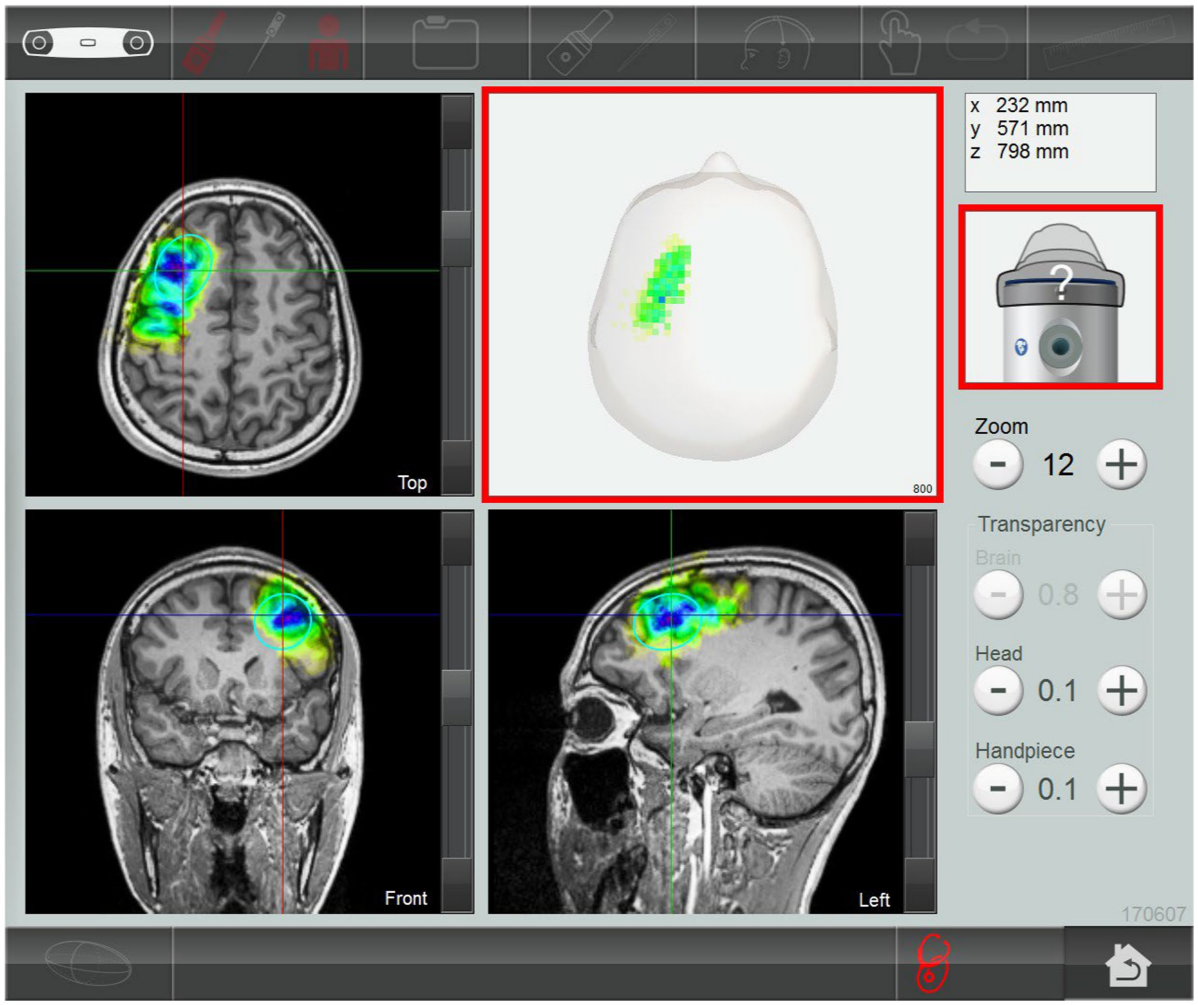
Subject’s MRI T1-weighted images. The stimulated treatment region (left dorsolateral prefrontal cortex) after transcranial pulse stimulation session.
